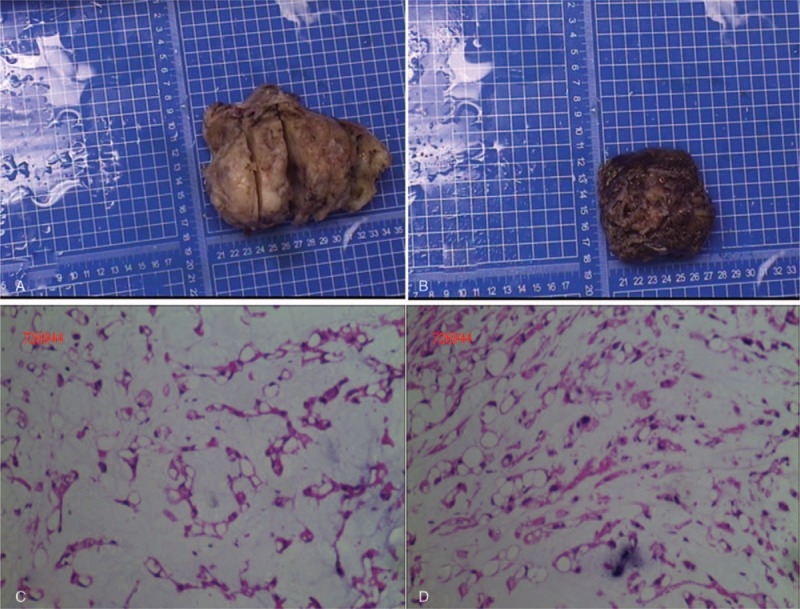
CT angiography did not suggest the blood supply of the mass.
